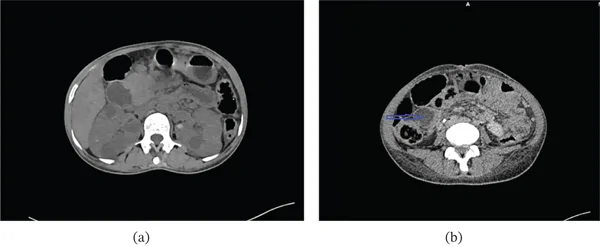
(A) Triphasic CT scan showing pancreatic body mass infiltration into the adjacent gastric wall. (B) Tumor thrombus in the portal vein at the splenic confluence, with direct hepatic invasion of the left lobe and multiple right hepatic metastases (see arrow).
